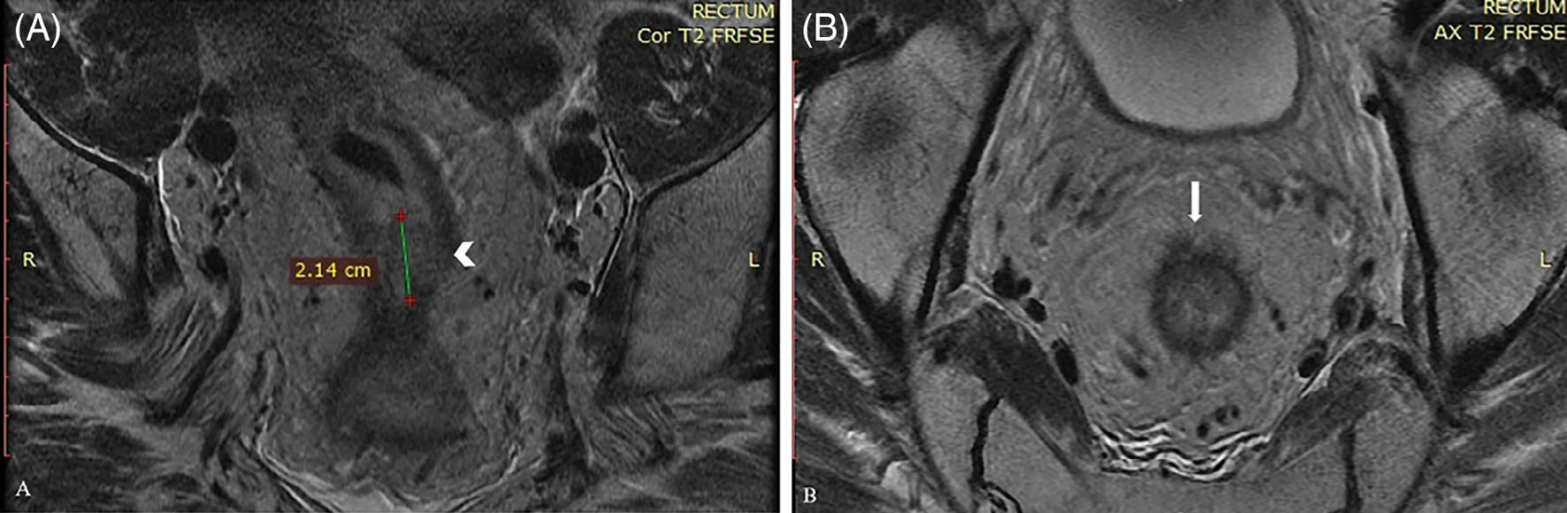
(A) After Neoadjuvant Radiotherapy Magnetic Resonance Imaging (MRI) (Protocol for rectal cancer). Coronal T2 sequence. A soft tissue (arrowhead) measuring 2.1 cm. (B) After Neoadjuvant Radiotherapy MRI (Protocol for rectal cancer). Axial T2, local blurring (arrow) of the perirectal tissue (extends <5 mm beyond muscularis propria, T3b). Mesorectal fascia and levetor ani muscles without signs of infiltration. Extramural Vascular Invasion (−). Lymph nodes without pathological findings
